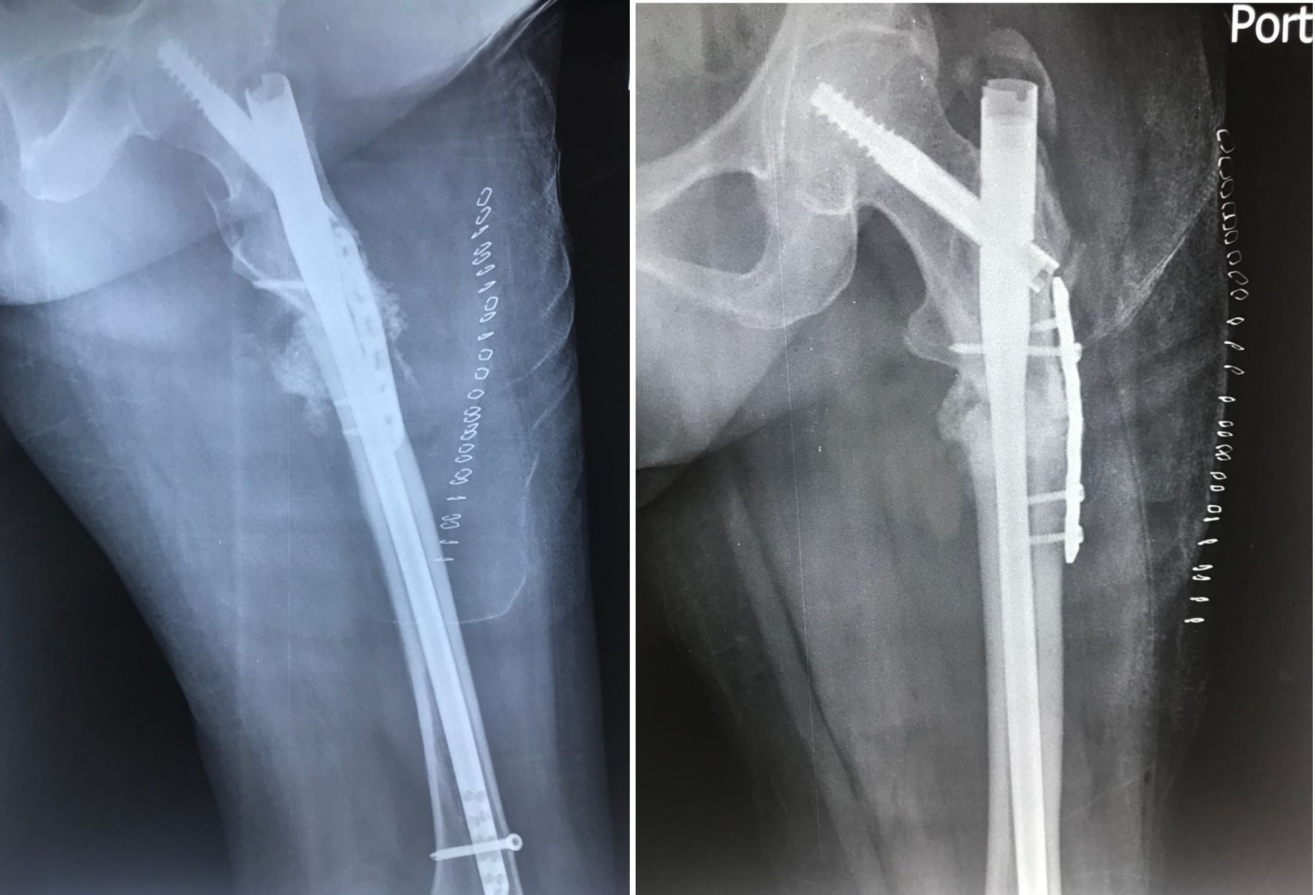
Immediate postoperative X-ray showing the augment plating done with bone grafting and bone marrow injection. Plating was done to provide compression at the fracture site.
